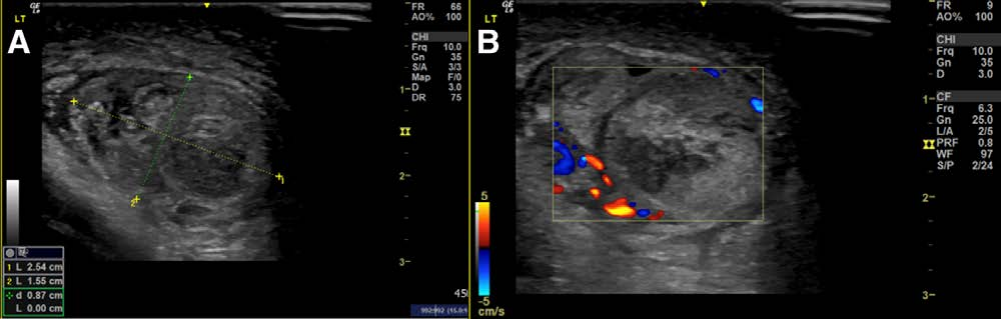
Doppler ultrasound of the left testicle. (A) Heterogenous content of left testicle and (B) no evidence of flow of left testicle.
